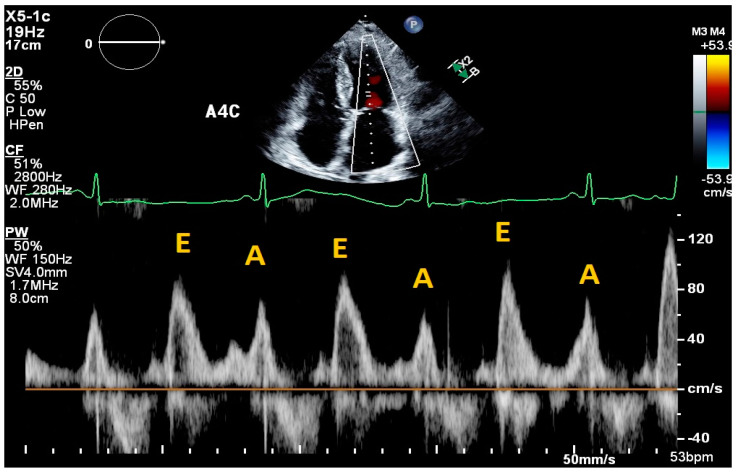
Mitral inflow velocities. Apical four chamber view. The pulse-wave Doppler sample volume is placed between the mitral valve leaflets at the level of the leaflet tips. Velocity tracing below. E wave corresponds to rapid ventricular filling. A wave corresponds to atrial systole.
